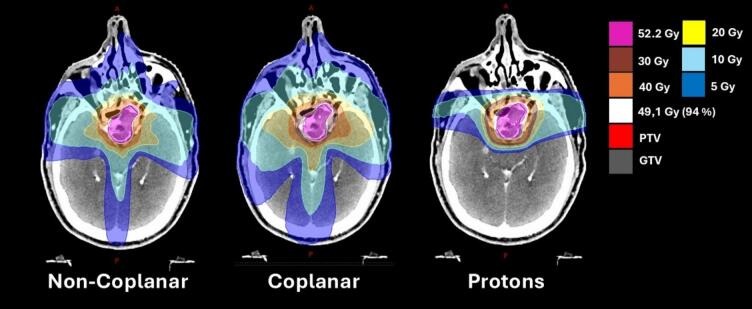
Dose colour-wash images with isodose lines overlaid on the planning CT scan. Dose levels are indicated in the top right. The GTV, PTV, and D_94%_ are also visualized.
